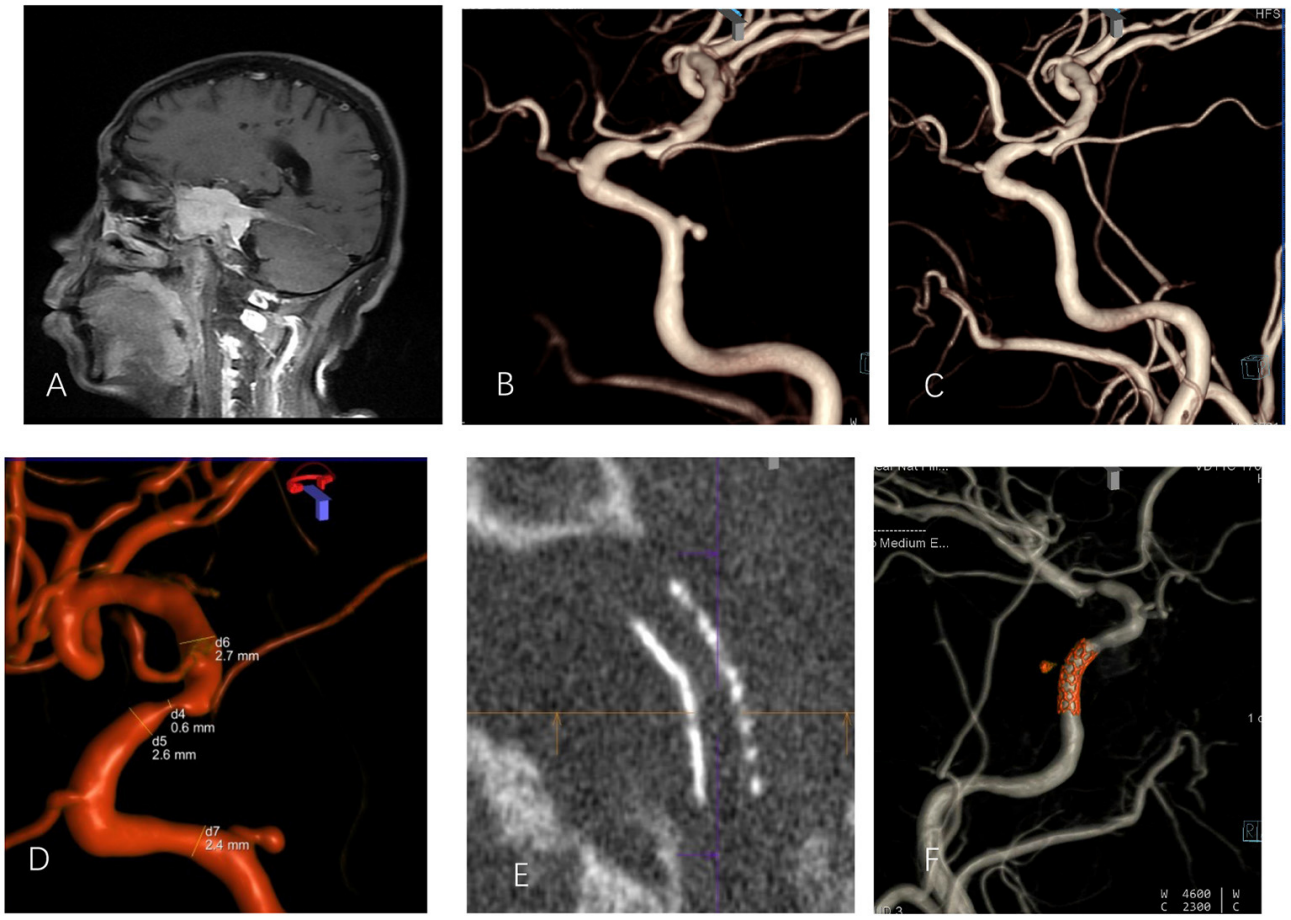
A 51-year-old male with intraoperative internal carotid artery injury of right sphenoid clival meningioma and postoperative secondary right internal carotid cavernous pseudoaneurysm before and after interventional therapy (A). Enhanced MRI revealed that the tumor wrapped the right internal carotid artery. (B) A DSA-3D lateral image showed pseudoaneurysm of the posterior wall of the cavernous sinus of the right internal carotid artery. (C) The pseudoaneurysm disappeared after stent treatment. (D) Measurement of the diameter of the parent artery; (E) Maximum intensity projection showed that the stent opened completely. (F) Postoperative dual-volume imaging showed that the stent graft had good compatibility and that the vessel was patent.
